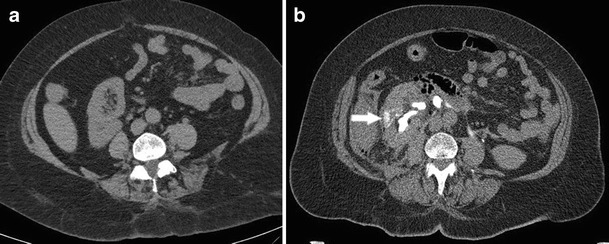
Eighty-year-old woman with gastric cancer mid-treatment with cisplatin and 5-FU. (a) Pretreatment axial CT demonstrating normal renal characteristics. (b) Noncontrast axial CT demonstrating contrast retention in the right renal parenchyma consistent with ATN (*arrow*)
